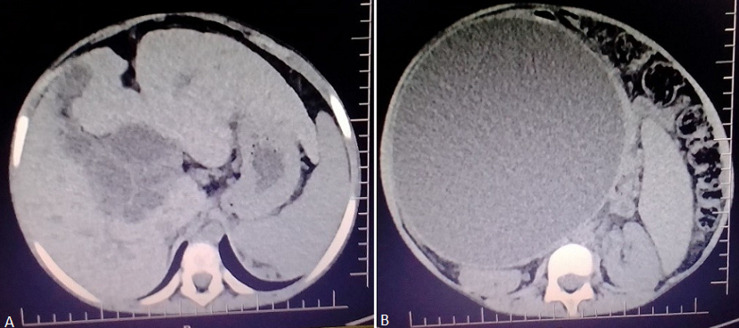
A, B) triphasic CT scan of the abdomen showed a well-defined retro-gastric cyst with dilatation of intra and extra biliary tree; differential diagnosis of choledochal cyst and pancreatic pseudocyst was made
